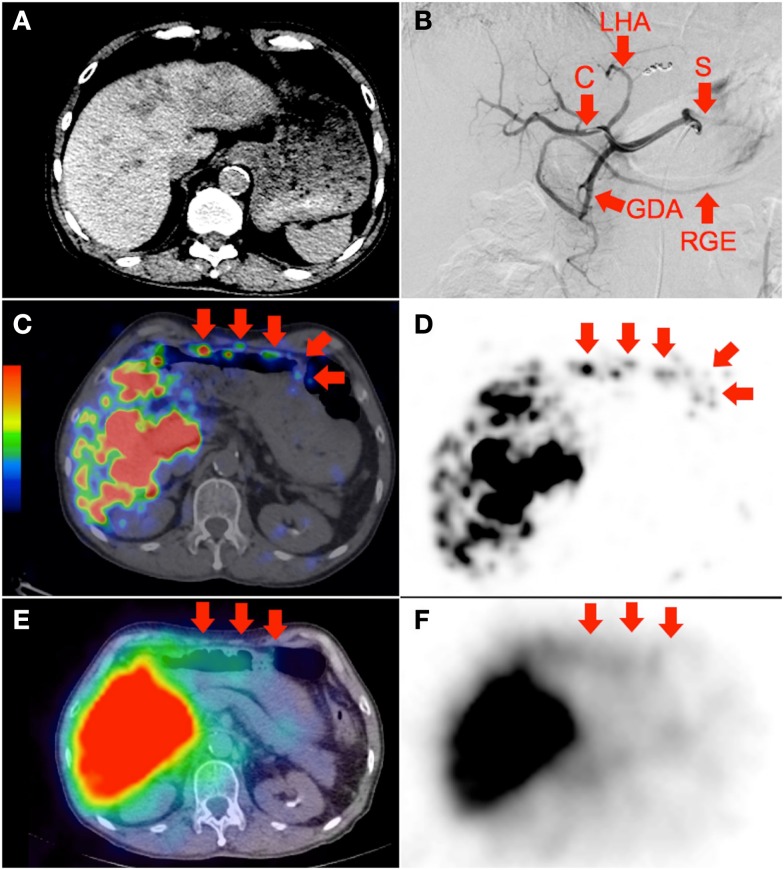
(A) Multifocal hepatocellular carcinoma with predominantly left lobe disease seen on non-contrast-enhanced CT. The patient was recently treated with sorafenib, a multi-kinase inhibitor with anti-angiogenic properties. RE was performed in two injections via the left and right hepatic arteries. Prophylactic coil embolization of the gastroduodenal artery was not performed at the discretion of the interventional radiologist. (B) Digital subtraction angiogram immediately after completion of right hepatic artery RE with the catheter tip (“C”) in the right hepatic artery demonstrates significant vascular stasis and reflux of contrast into the left hepatic (“LHA”), proper hepatic, gastroduodenal (“GDA”), right gastroepiploic (“RGE”), common hepatic and splenic (“S”) arteries. (C,D)
